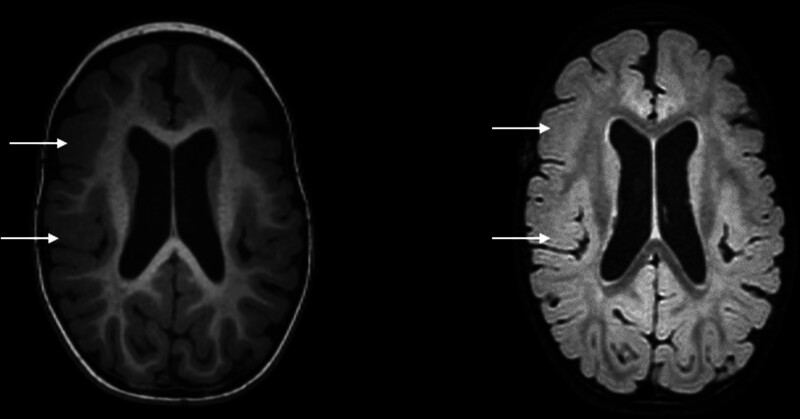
Cranial MRI of the proband (left, T1WI image; r, T2 flair image) revealed reduced medullary volume, fewer gyri, and focal cortical thickening in both hemispheres, indicating MCD. MCD = malformations of cortical development, MRI = magnetic resonance imaging.
